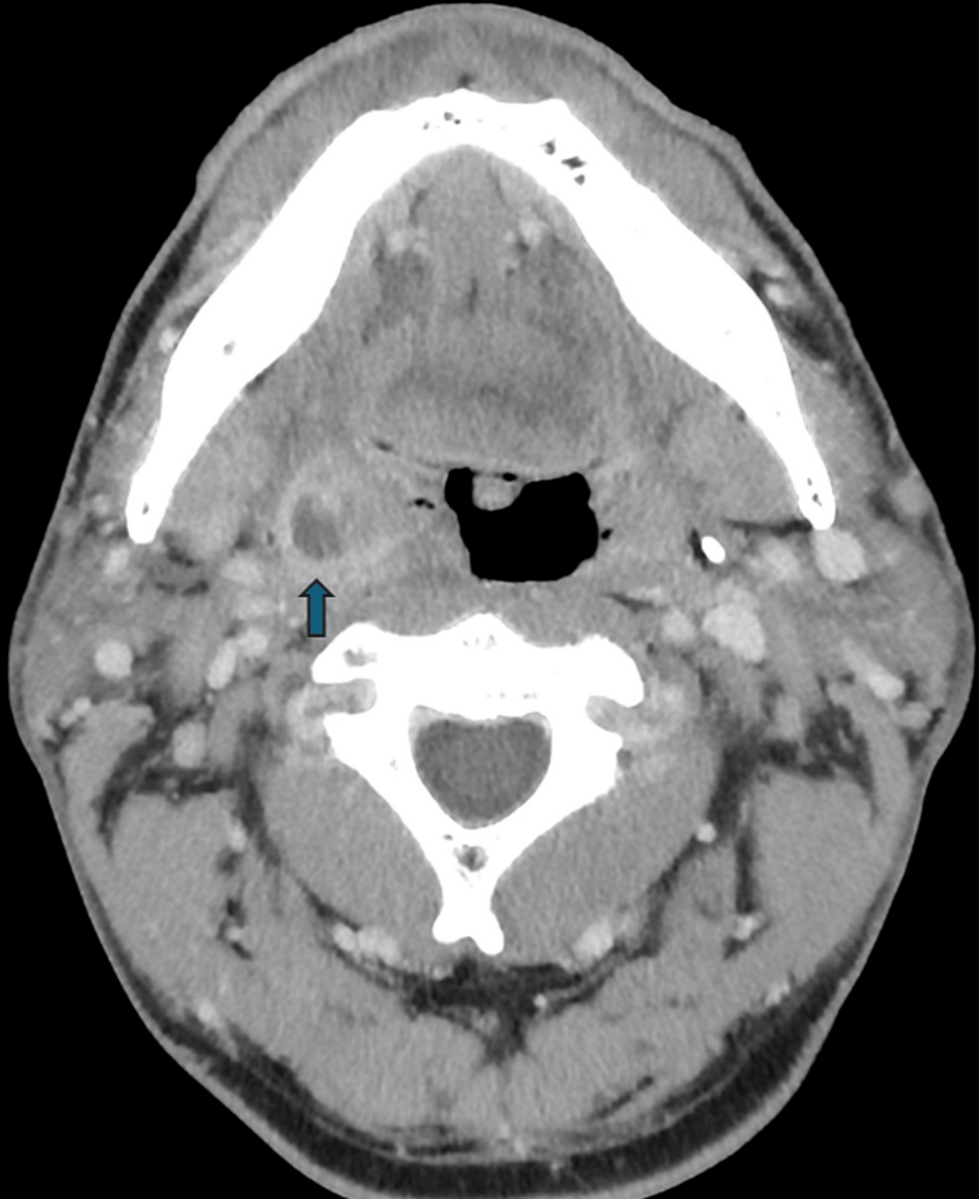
Contrast-enhanced CT image The image shows a right peritonsillar abscess approximately 1 cm in size observed in the right tonsil (blue arrow).
